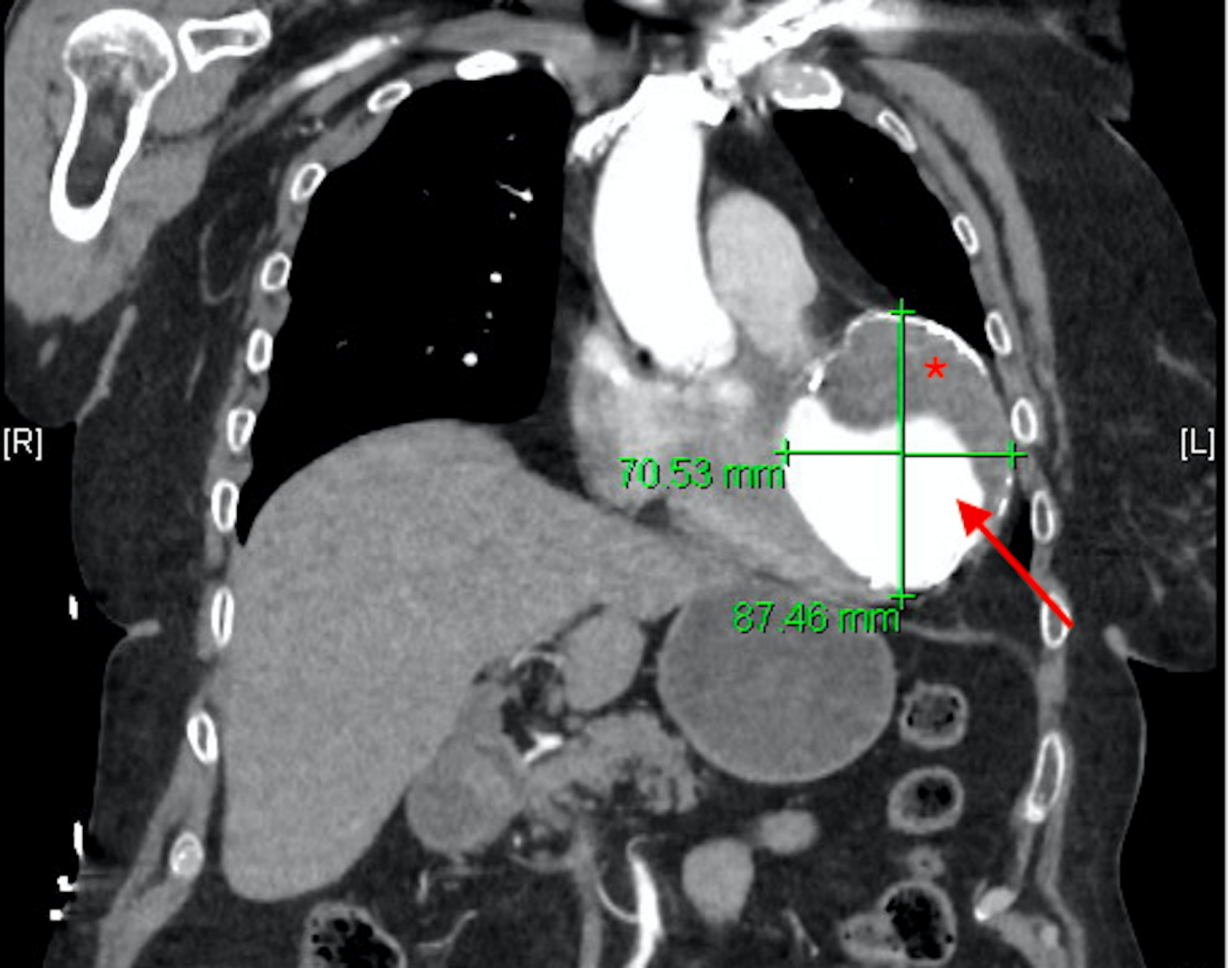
Contrast-enhanced CT, coronal section, shows a large opacity seen connected with the left ventricle extending down near the left hemidiaphragm (arrow). A mural thrombus is also seen within the aneurysm (asterisk).
